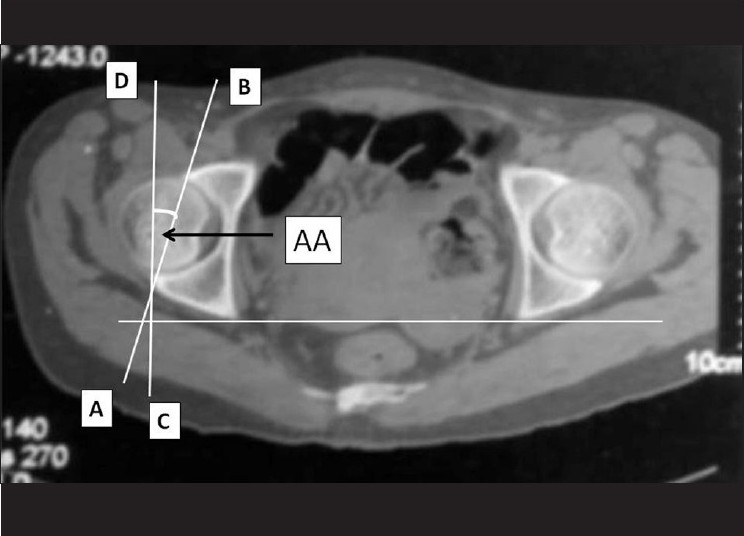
On an axial CT scan through the center of the acetabulum, a line was drawn between the anterior and posterior edges of the acetabulum (AB) and the angle between this line and a plane sagittal to the pelvis (CD) was determined to be acetabular anteversion
